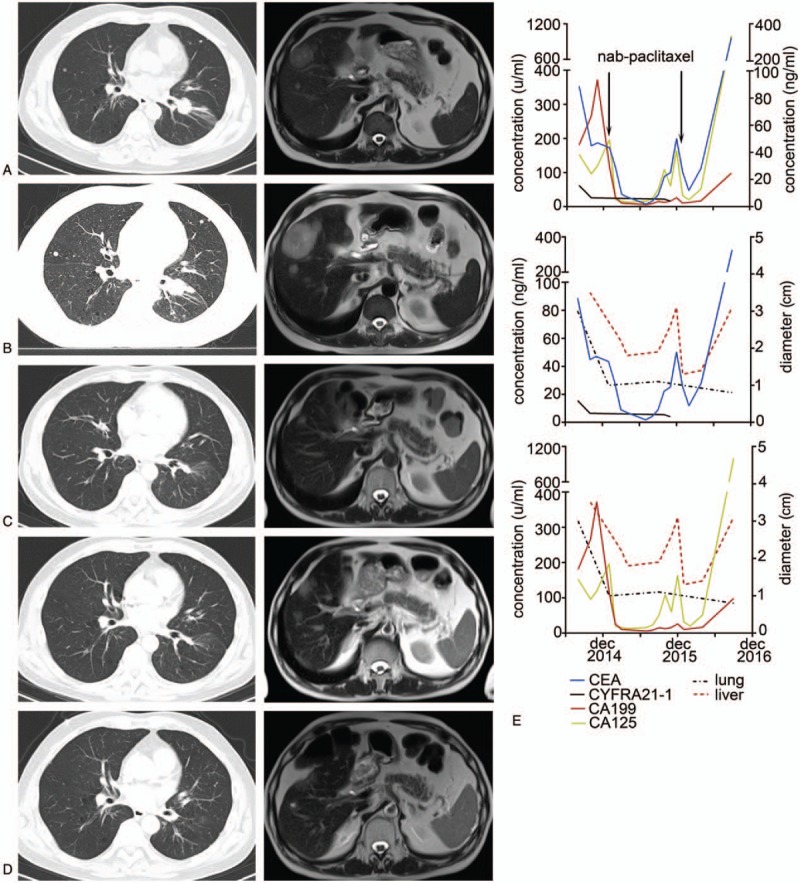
(A) After nedaplatin–pemetrexed chemotherapy, CT and magnetic resonance imaging in November 2014 before gefitinib treatment, implied lung cancer in left lower lobe and metastases in liver. (B) After treatment with gefitinib, lung masses on CT scan in January 2015 were stable, but metastases in liver progressed. (C) The patient achieved nearly complete remission in lung and partial remission in liver. (D) Upper panels: progression in liver in September 2015, lower panels: partial remission after treatment with paclitaxel–avastin and erlotinib, lesions in liver shrunk. (E) Dynamic changes of serum tumor markers including CEA, CYFRA21-1, CA199, and CA125, as well as the diameters of tumors in lung and liver are shown. CT = computed tomography.
